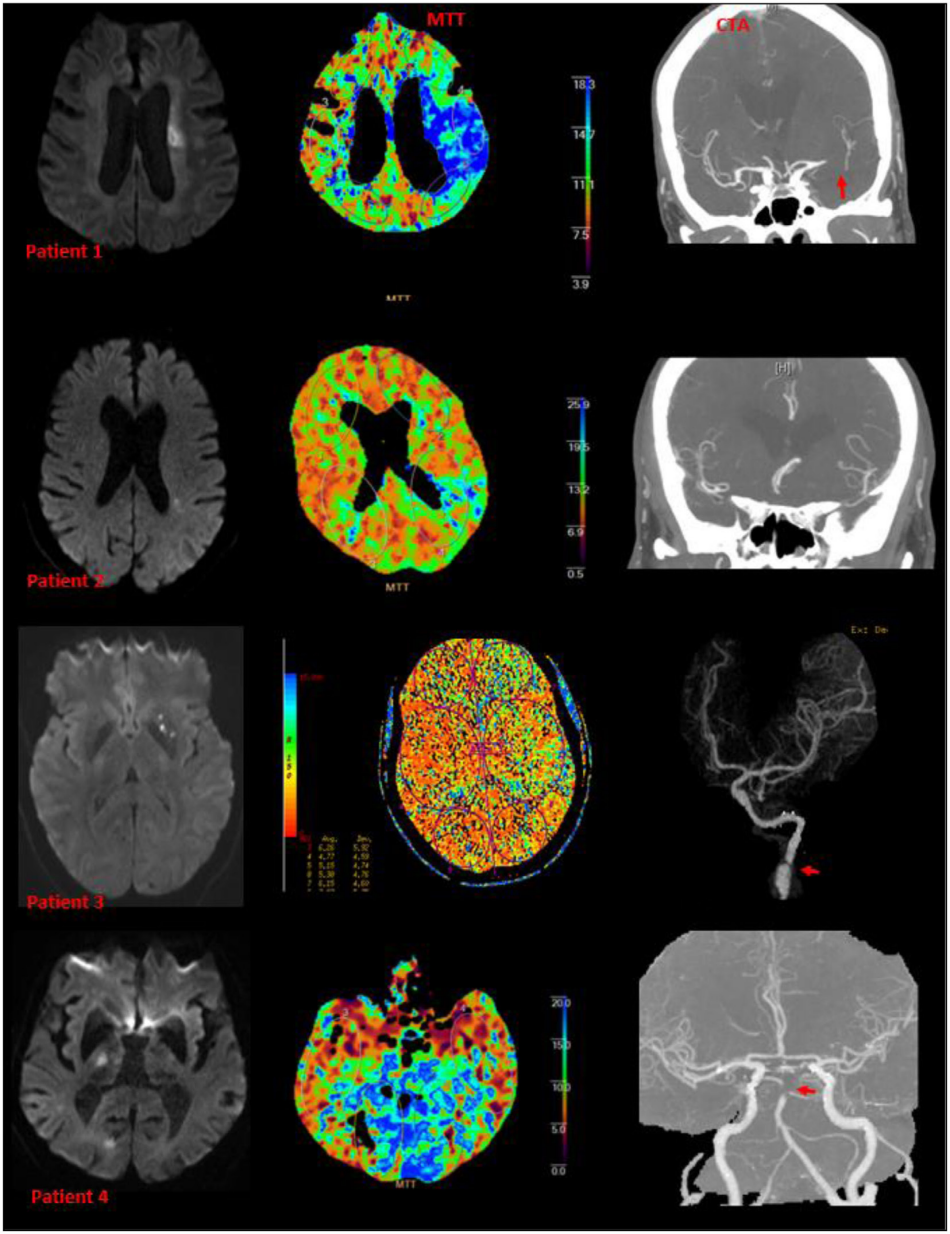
Examples of small subcortical infarct, perfusion deficit, and large artery diseases. Patients 1, 3, and 4 have abnormalities on CT angiography (patient 3 had arterial dissection of the internal carotid artery). Patient 2 had M3 branch occlusion on CT angiography.
